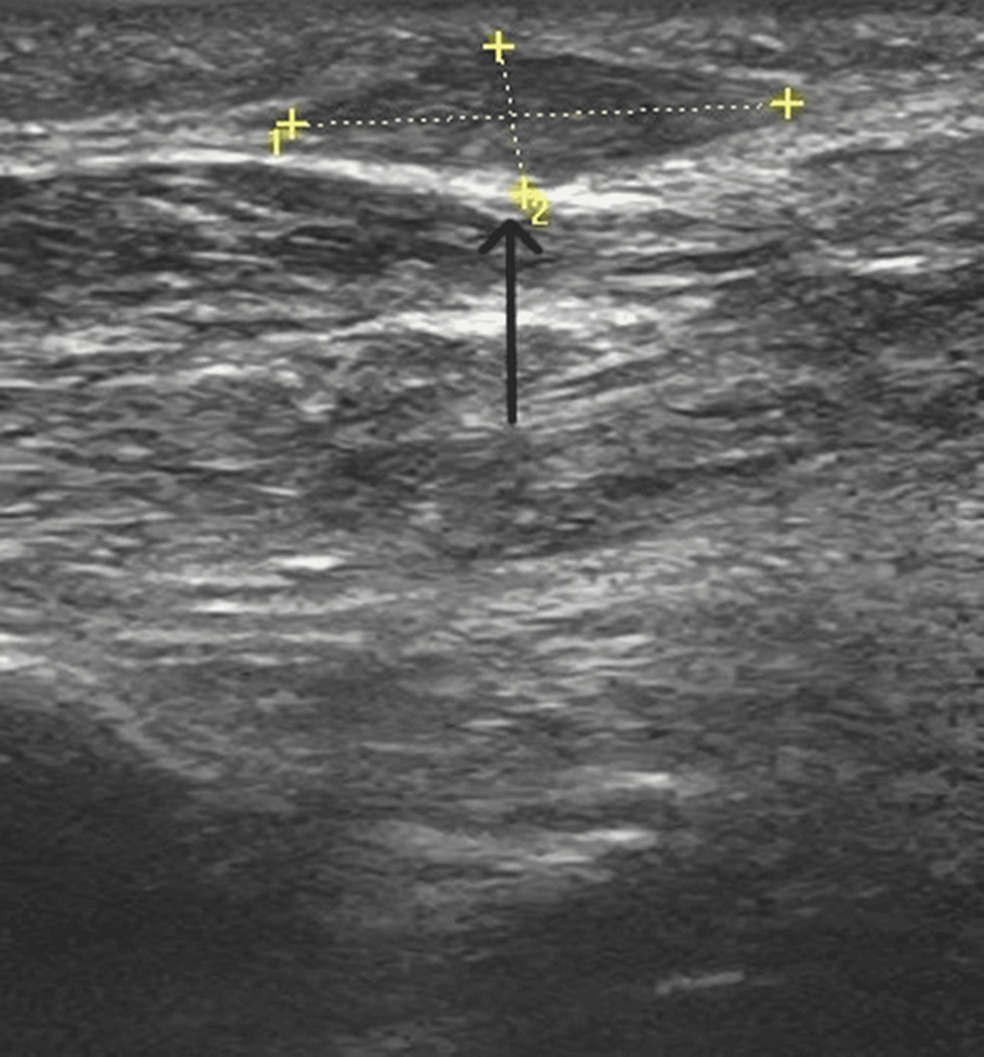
Ultrasound of the left foot revealed a nodule measuring approximately 1.40 cm in its longest axis and 0.50 cm in its shortest axis.
